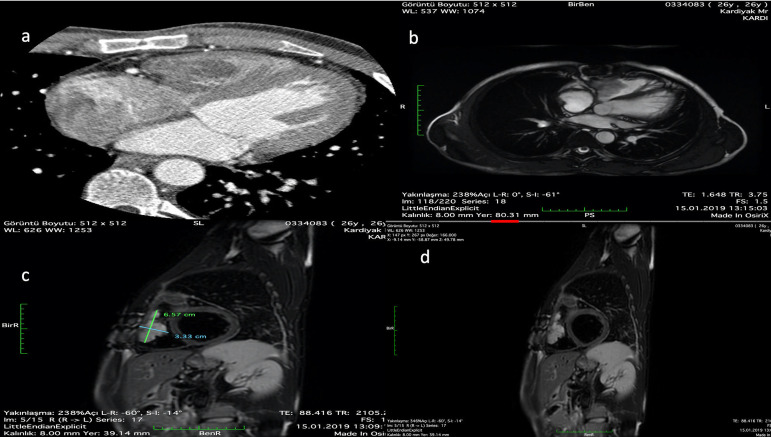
(A) Computed tomography shows right ventricular myxoma that is connected to right ventricular basal free wall. (B) Four-chamber MRI view shows right ventricular myxoma that is connected to the right ventricular basal free wall. (C) MRI short-axis view shows the measure of the myxoma in 6,57×3,33 centimeters. (D) Gadolinium-enhanced MRI imaging revealed heterogeneous delayed enhancement of the RV mass confirming the diagnosis of myxoma.
